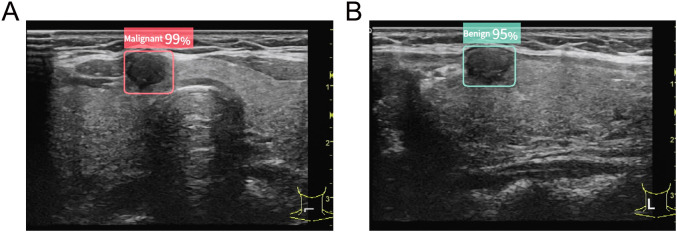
Representative case of discordant AI output between transverse and longitudinal planes. (A) Transverse-plane and (B) longitudinal-plane ultrasound images of a thyroid nodule in a 45-year-old man show a higher malignant-risk output on the transverse plane than on the longitudinal plane. According to the predefined rule, the more suspicious result was retained, and the final AI classification was “Possible Malignant.” Postoperative pathology confirmed papillary thyroid carcinoma.
